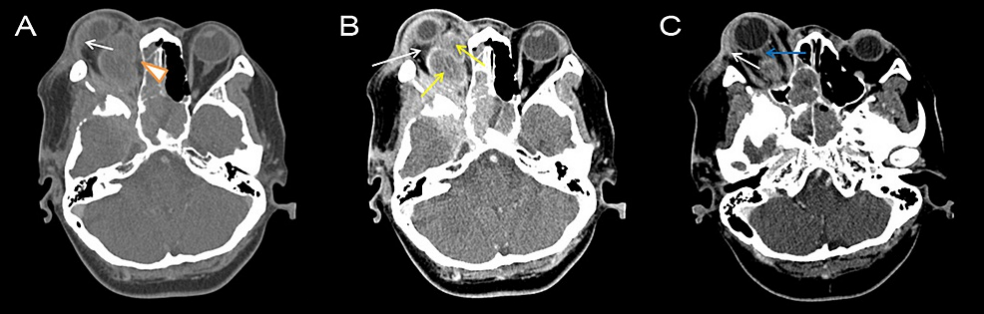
Axial post contrast computed tomography image demonstrates intraorbital extension of sphenoid wing meningioma with intraorbital fat stranding (white arrows), right optic nerve thickening (blue arrow) and two oval hypodense regions with rim enhancement, representing collections (yellow arrows). Arrowhead shows the location of possible right subperiosteal abscess.
